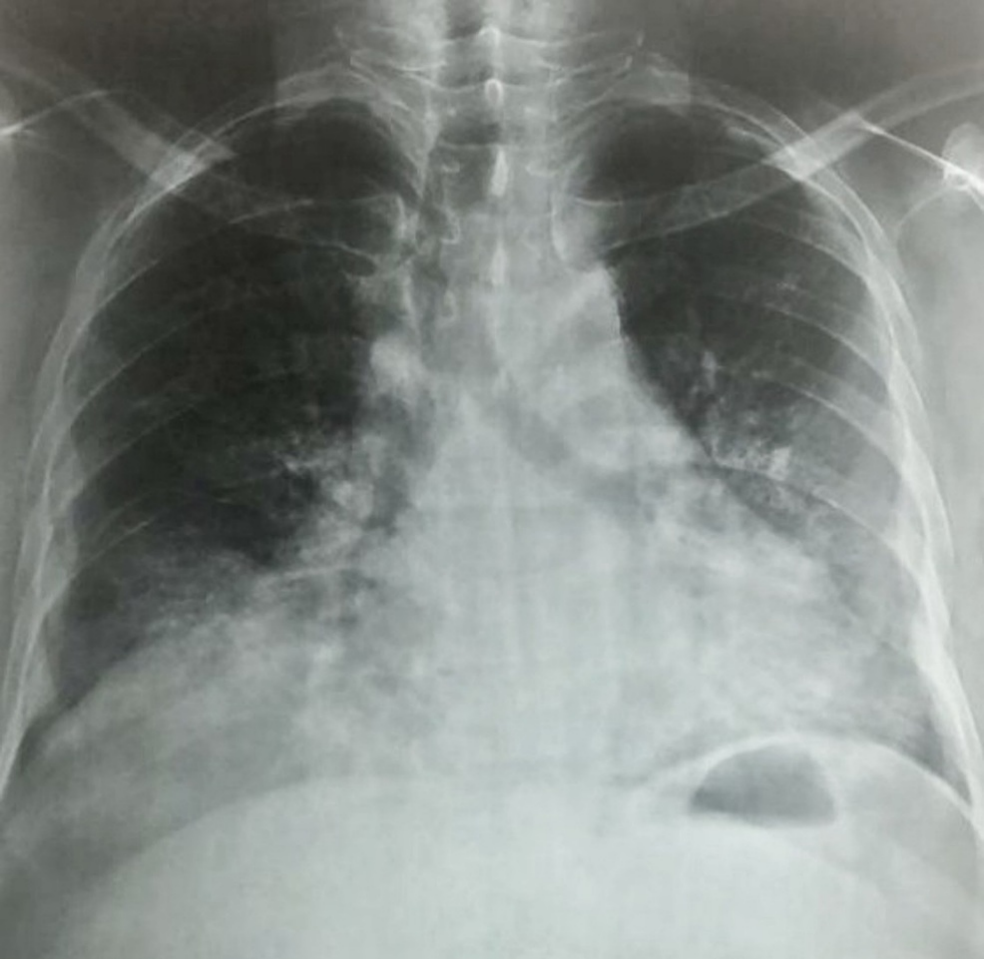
Subsequent chest X-ray of the patient demonstrating consolidation in the left middle zone and bilateral lower zone
